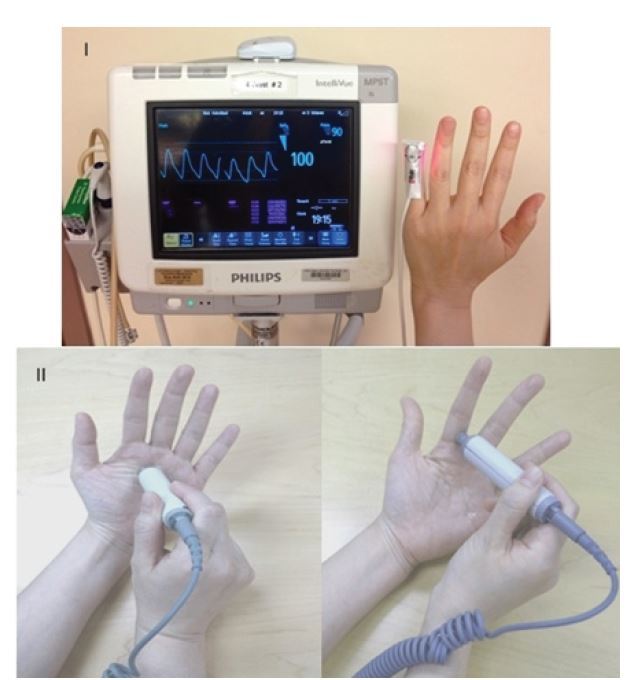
I: Digital pulse oximetry measurement; II: Doppler interrogation of palmar arch and digital artery.
